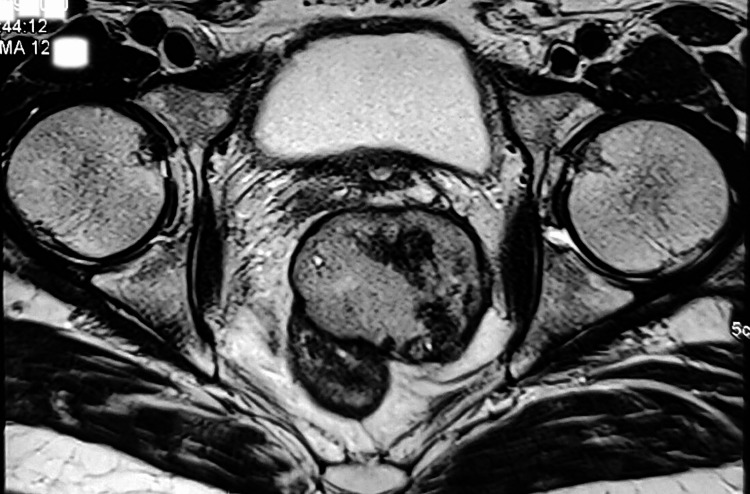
MRI scan on the first follow-up showing significant reduction in size
